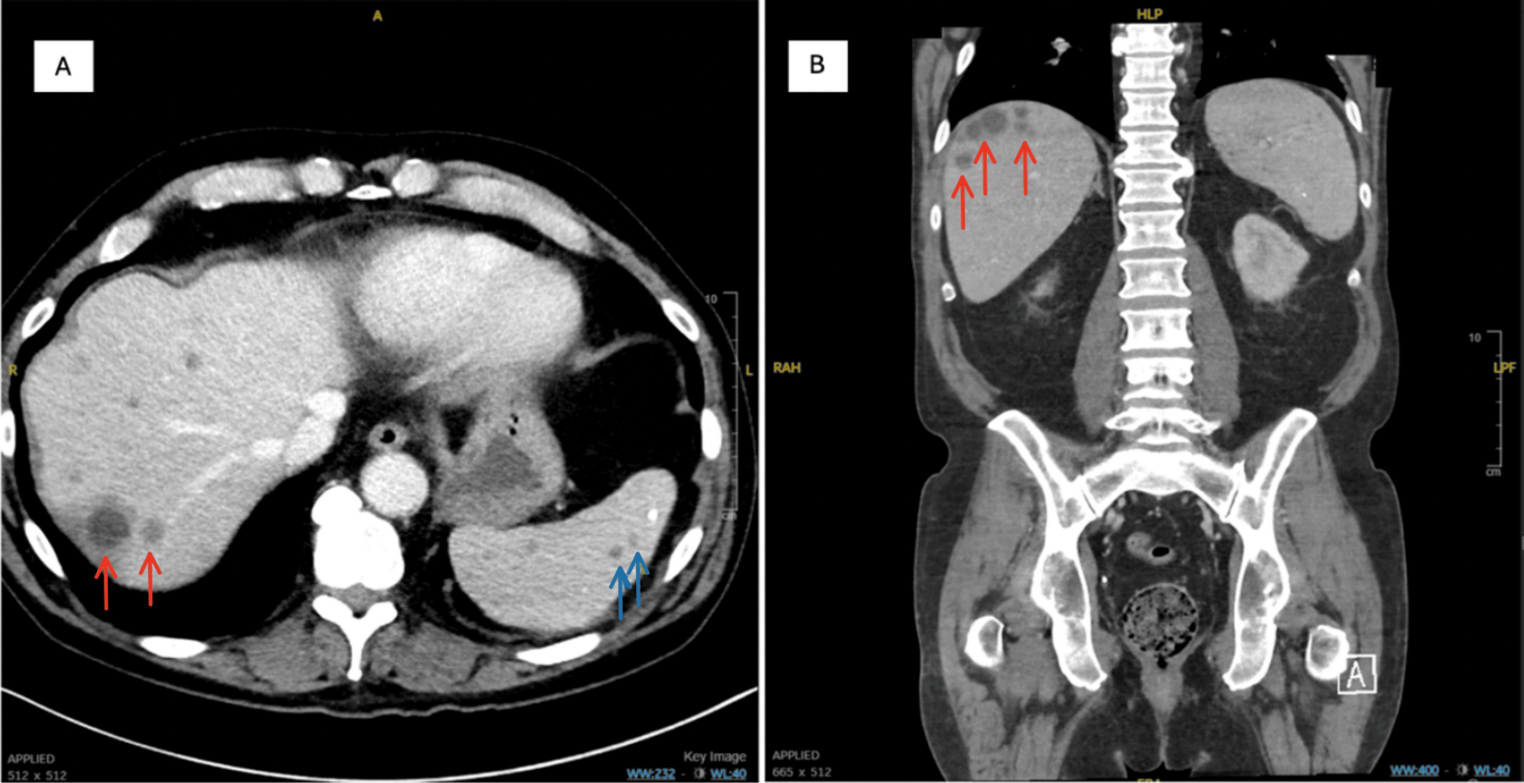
Imaging performed upon patient's initial presentation showing multiple hypoenhancing lesions of the liver (red arrows) and the spleen (blue arrows). CT of the abdomen and pelvis with contrast in axial view (A) and coronal view (B).
